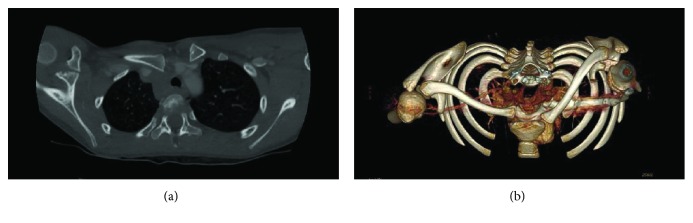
Axial (a) and three-dimensional reconstructed (b) computed tomography scans confirm the posteriorly displaced clavicle on the right side. Due to the absence of the corresponding centers of ossification of the medial clavicular epiphysis, discrimination from a pure posterior dislocation of the sternoclavicular joint is difficult.
